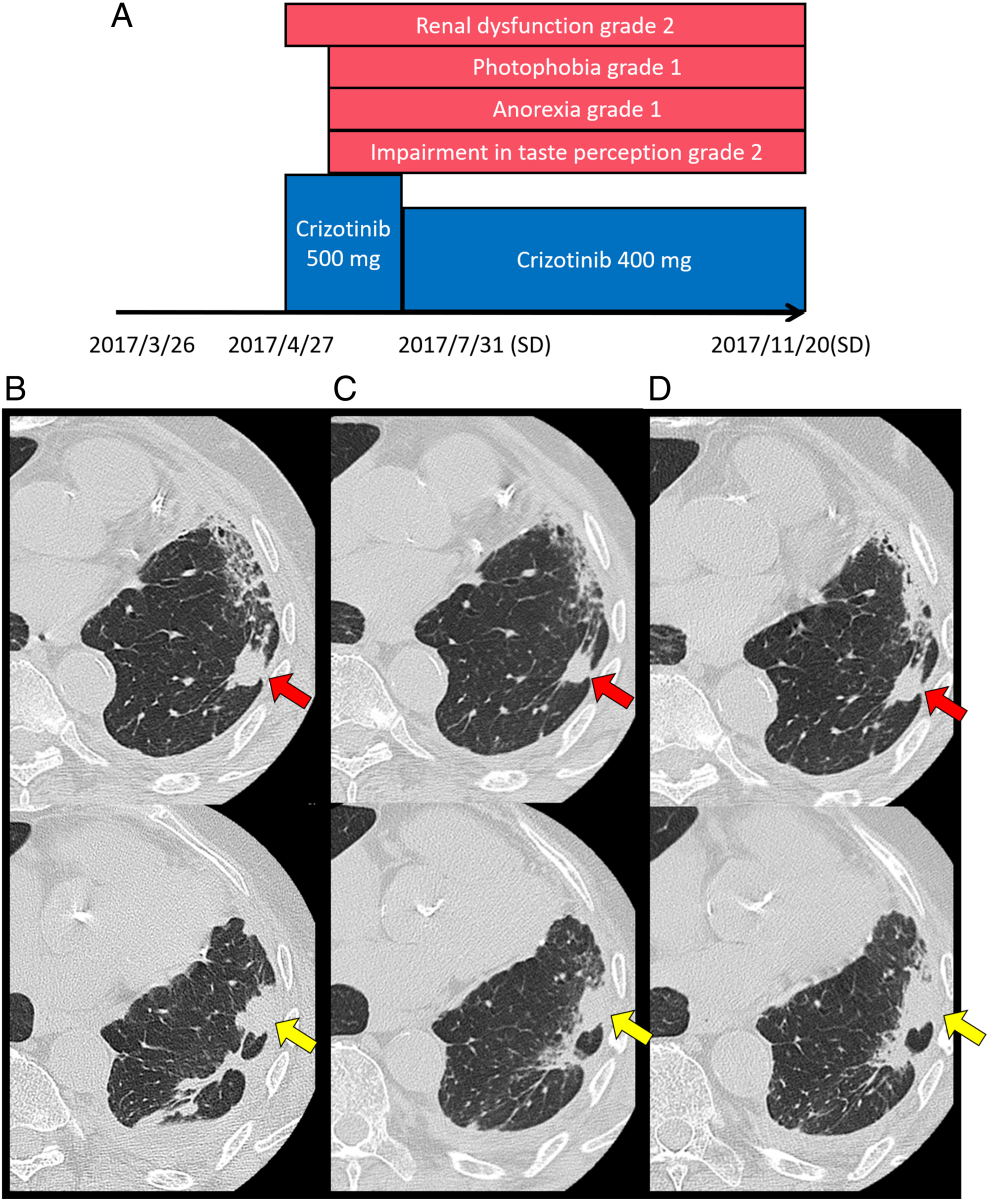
Clinical course after administration of crizotinib. (A) Clinical course and adverse events were presented after administration of crizotinib. (B) CT scan of the chest showing nodular lesions in the left lower lobe (red arrow, 15 × 15 mm; yellow arrow, 20 × 15 mm) after the initiation of crizotinib on 26 March 2017. (C) Both target lesions (red arrow, 12 × 11 mm; yellow arrow, 16 × 13 mm) decreased slightly on 31 July 2017. (D) Both target lesions (red arrow, 14 × 13 mm; yellow arrow, 20 × 15 mm) increased slightly on 20 November 2017.
